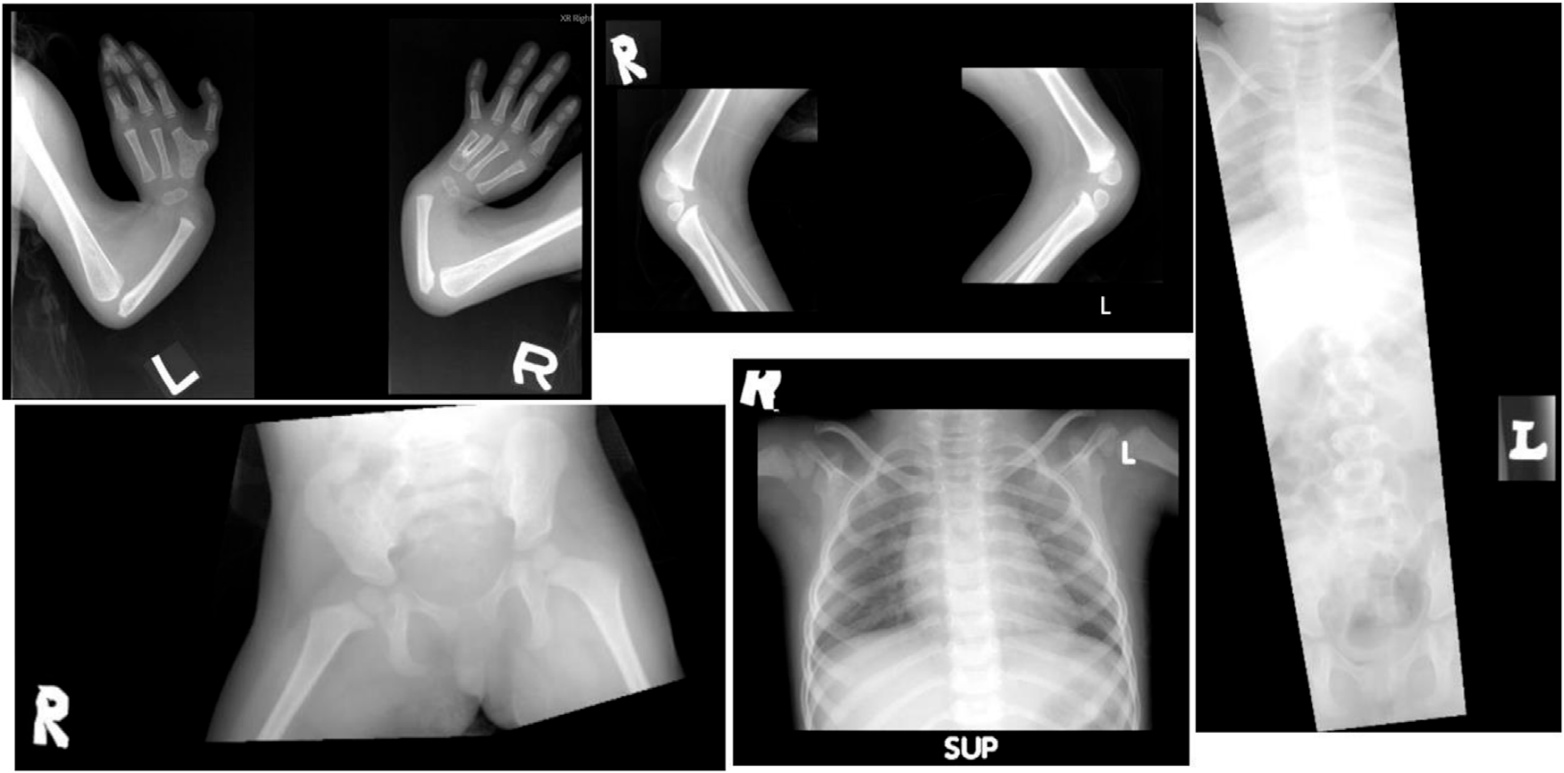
Patient’s radiograph at the age of 2 years old. There was a shortened ulnar, with the absence of radius bilaterally, and fusion of the left metacarpal bone. The spine and pelvic bone appeared normal.
